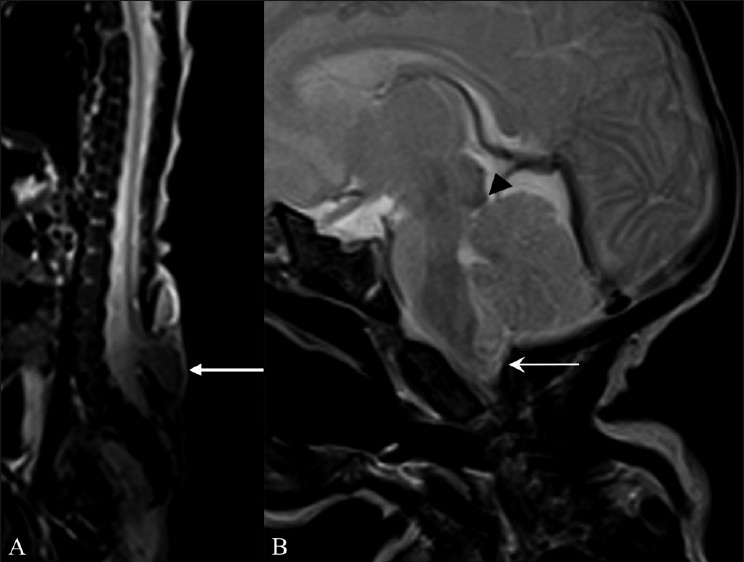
Sagittal T2W whole spine MRI (A) shows a meningomyelocele (arrow) opposite the L5 and S1 vertebrae. Sagittal T2W MRI of the brain (B) shows a small posterior cranial fossa, with herniation of the cerebellar vermis and tonsils (arrow) through the foramen magnum with tectal beaking (arrowhead). These findings are suggestive of an Arnold–Chiari II malformation
